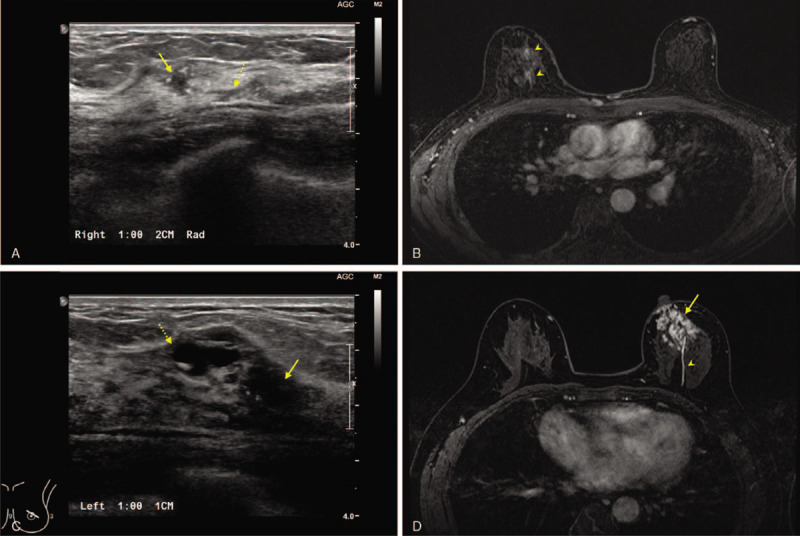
Preoperative findings of the two patients. (A, B) Patient 1 was diagnosed with an invasive ductal carcinoma in her right breast. Ultrasonography revealed a 0.5-cm irregular mass (arrow). Duct dilatation (dotted arrow) was extended at the end of the main lesion. Two different, enhanced lesions (arrowheads) were identified in preoperative breast magnetic resonance imaging (MRI), which correlated with sonographic findings. The total clinical tumor size was 2.6 cm shown on breast MRI. (C, D) Patient 2 was diagnosed with an invasive ductal carcinoma in the periareolar region of her left breast. Ultrasonography detected a 1.2-cm main mass (arrow). A suspicious cystic lesion (dotted arrow) was also identified in the medial side of the main lesion. A total extent of 4.9 cm of the enhanced lump (arrow) was found in the subareolar region of the left breast, and one enhanced duct (arrowhead) from the mass was also verified.
